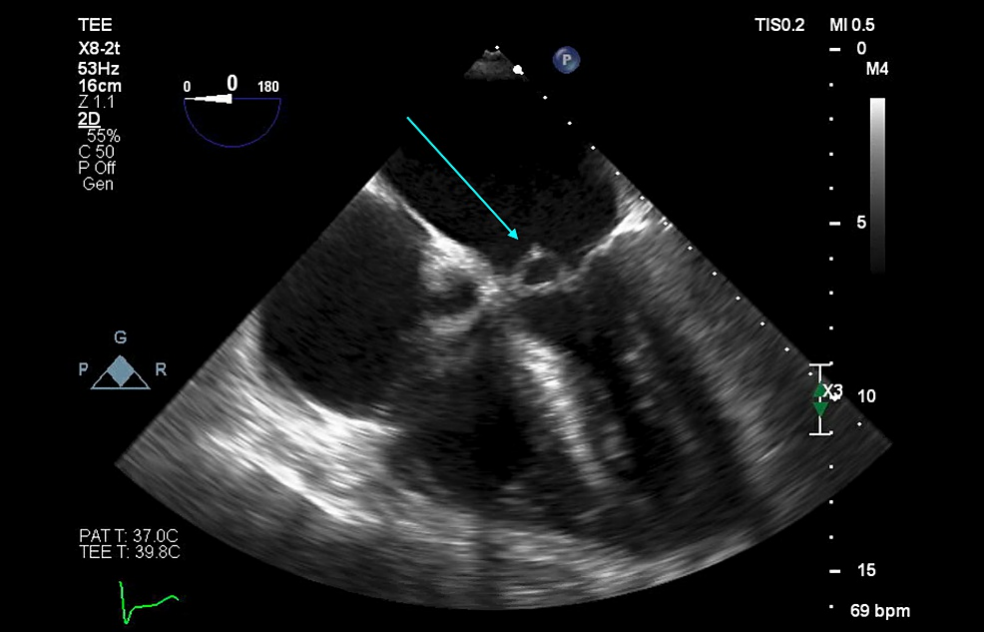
Mid-esophageal four-chamber view of transesophageal echocardiogram showing vegetation on the closed mitral valve (blue arrow)
